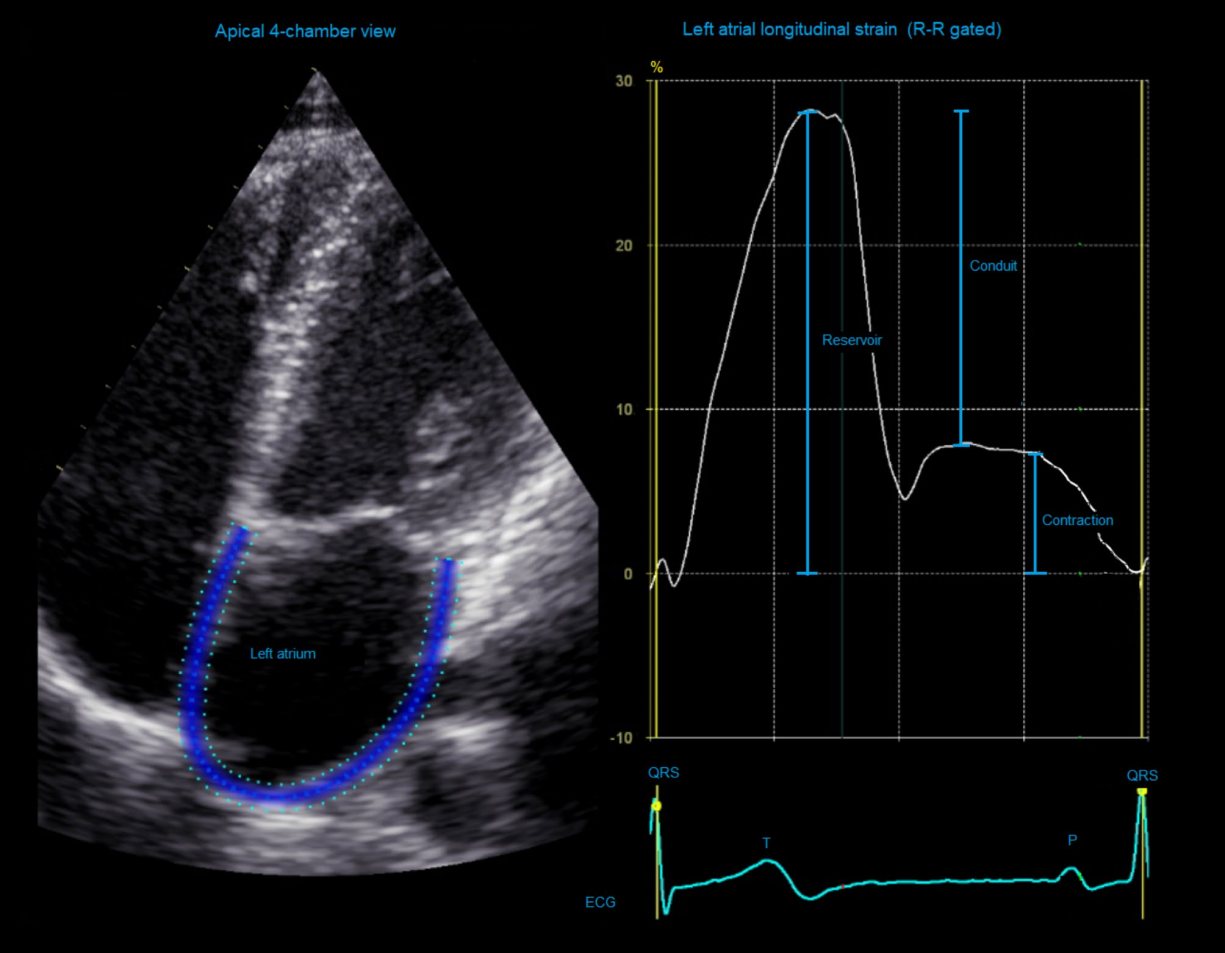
Left atrial reservoir, conduit, and contractile function measured by left atrial longitudinal strain in an apical 4-chamber view image
